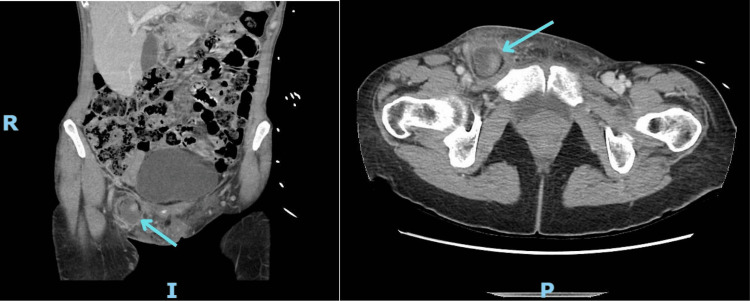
Coronal and axial cuts of the femoral hernia visualized on computed tomography (CT) scan of the patient
